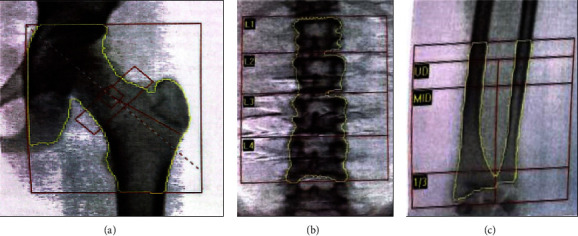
Dual energy X-ray images show low bone mineral density over (a) neck of femur, (b) lumber spine, and (c) distal radius.
